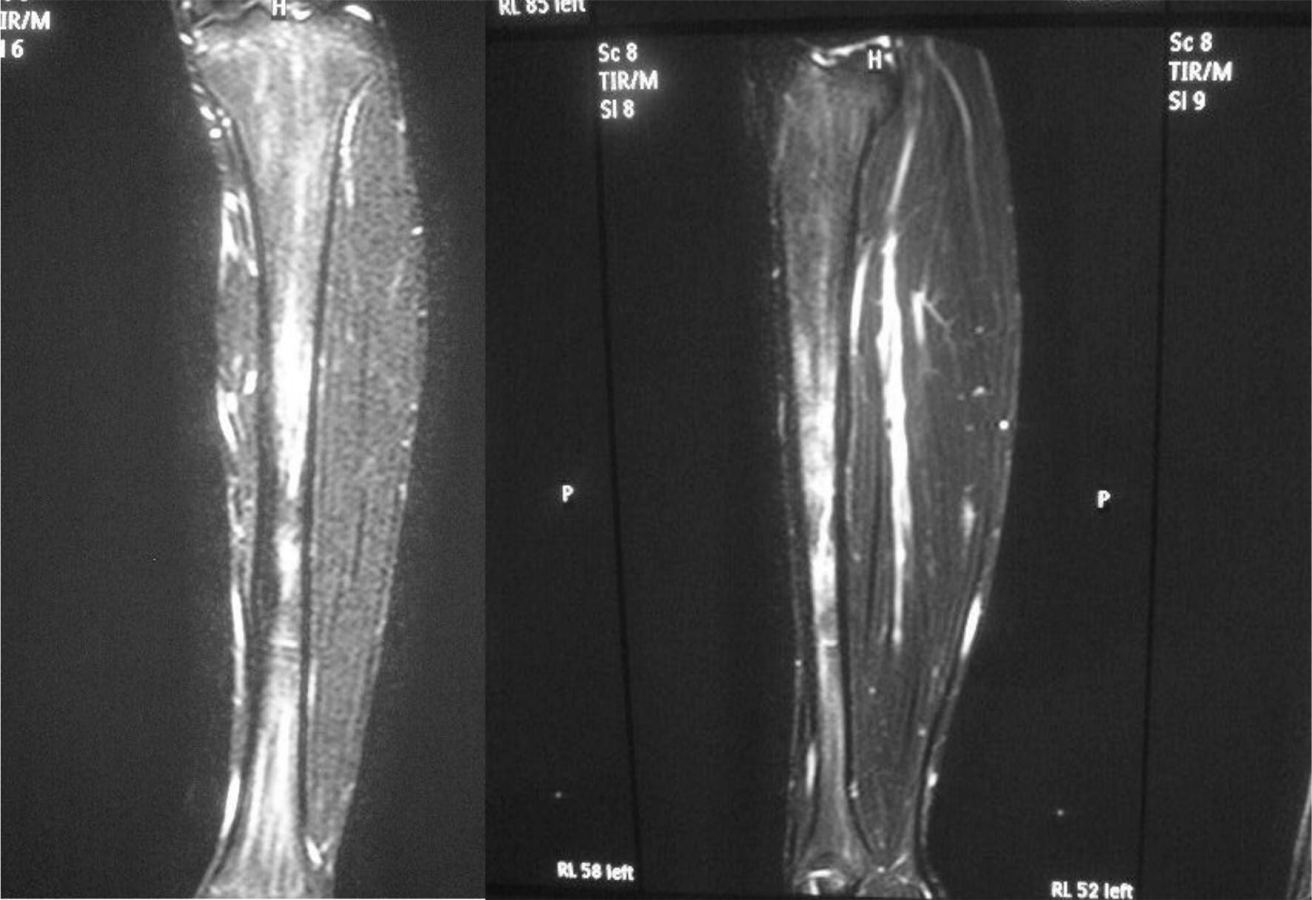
MRI of the left lower leg after right complete fractures. On MRI, STIR shows an abnormal high signal
